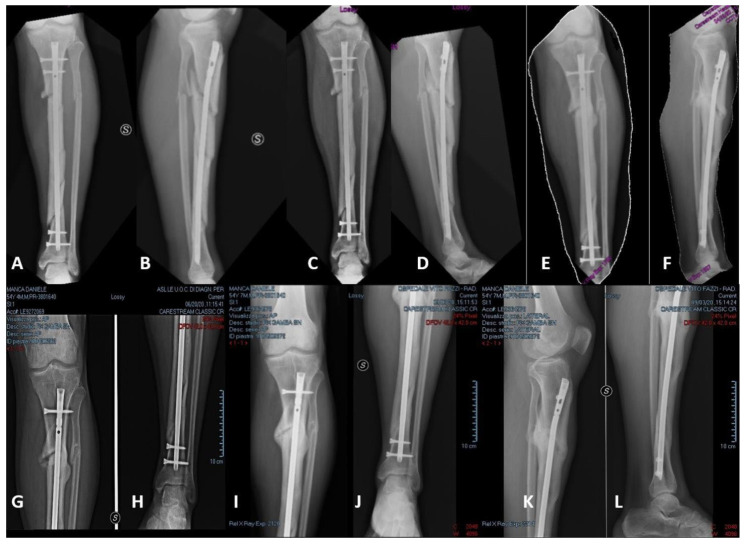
(A,B) A 49-year-old male after a vehicle accident with multisegmented tibial fractures treated by external fixation and nonunion with reamed nailing. (C,D) Aseptic nonunion after 3 months of tibia; (E,F) 1-month X-rays following Teriparatide injection. (G,H) Three months after the operation, X-rays revealed a bone callus on the third cortex. (I,J) At four months, X-rays revealed a healthy bone callus at the time of nailing dynamization. (K,L) At six months, X-rays revealed a hypertrophic proximal bone callus.
